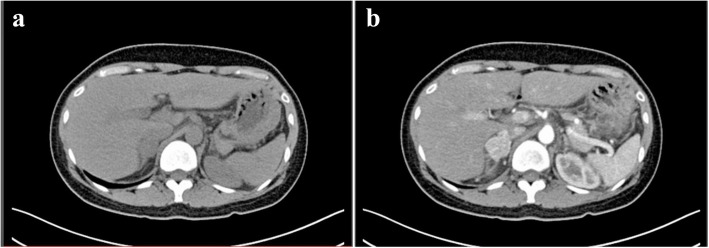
Computed tomography image showing a circular soft tissue density shadow in the right adrenal gland of the patient (a). The lesion in the arterial phase is markedly heterogeneous with a clear boundary after enhancement (b)
